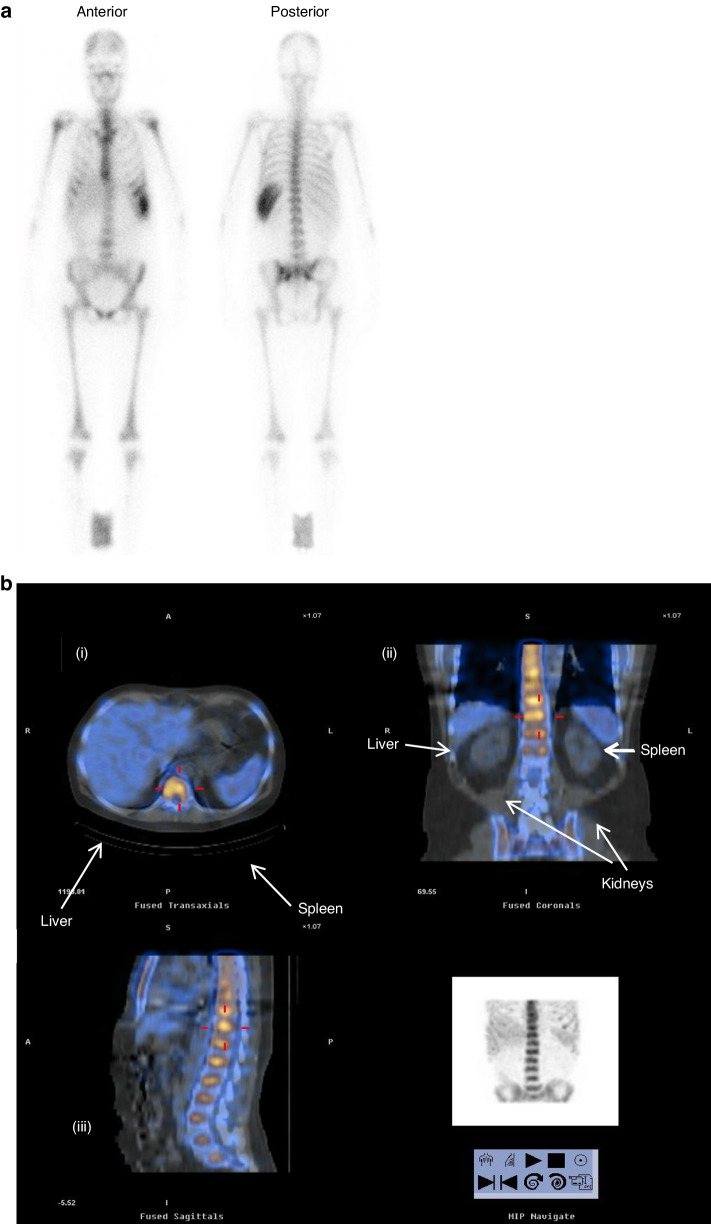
Planar and SPECT-CT gamma camera images post ^111^In-antiCD66 infusion. a Whole-body gamma-camera image showing distribution of ^111^In-anti-CD66 monoclonal antibody. b Superimposed SPECT gamma radiation (coloured) and CT images (grey scale). (i) Cross-section showing strong gamma radiation signal in L3 vertebral body (yellow), low in liver and spleen (blue) and undetectable in muscle, bowel. (ii) Coronal section image showing strong gamma radiation signal in vertebrae and pelvis (yellow), low in liver and spleen (blue) and very low in kidneys (grey scale image of CT). (iii) Sagittal image showing high gamma signal (yellow) in vertebral bodies, sternum and virtually none in other organs.
